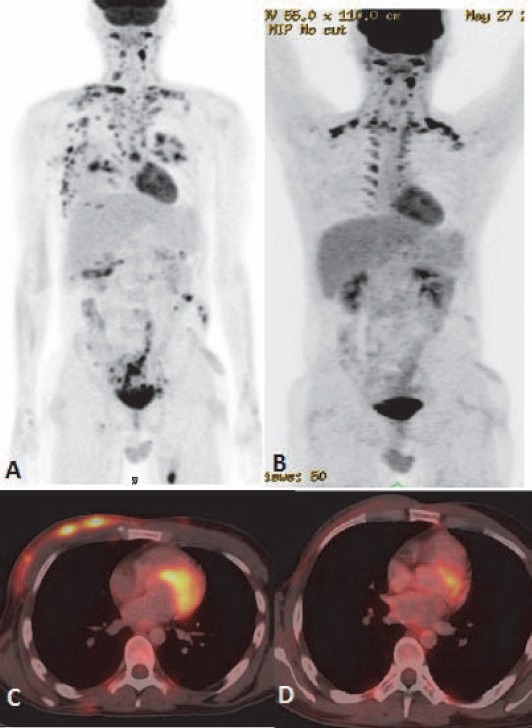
Cutaneous T cell lymphoma: (A) MIP image showing widespread focal radio tracer uptake in chest wall. (C) Corresponding fused PET/CT images shows FDG avid subcutaneous deposits in anterior chest wall. (D)Post therapy scan shows complete disappearance of the lesions. (B) MIP image of post therapy scan shows FDG uptake by brown fat
